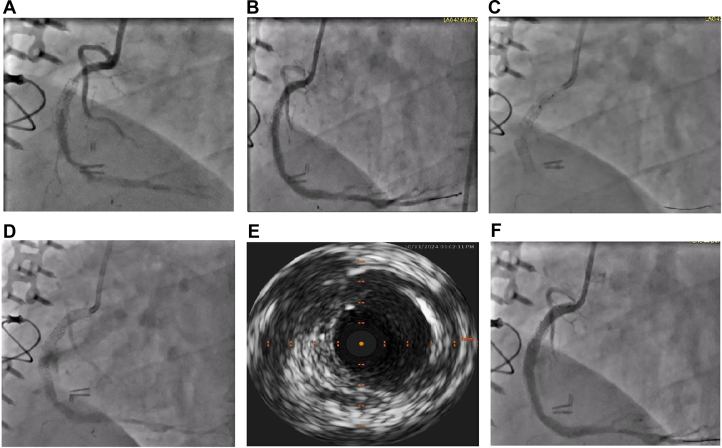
Coronary Angiogram of the Right Coronary Artery (A) Preintervention image; (B) postballoon dilatation and brachytherapy image; (C) stent IV fracture; (D) perforation; (E) intravascular ultrasound image showing perforation with stent gap; and (F) covered stent placement.
